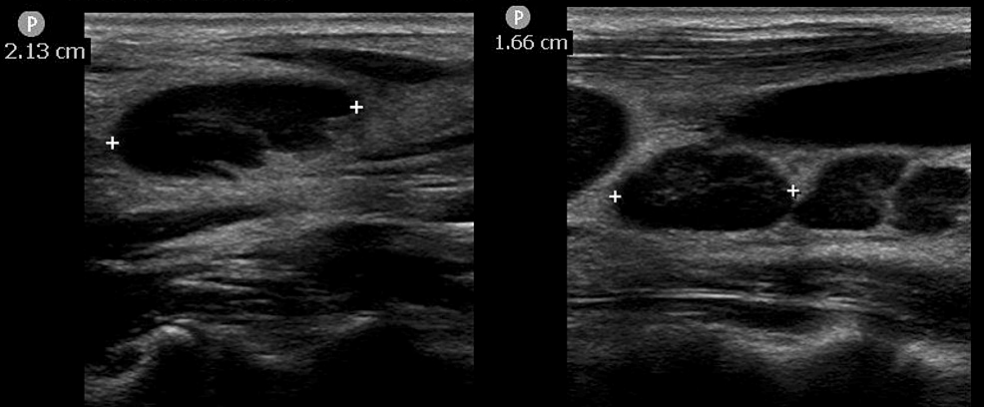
Ultrasonographic imaging of the right cervical lymph nodes in case 2 Multiple lymph nodes less than 25 mm with preserved hilum, suggesting reactive lymphadenopathy.
